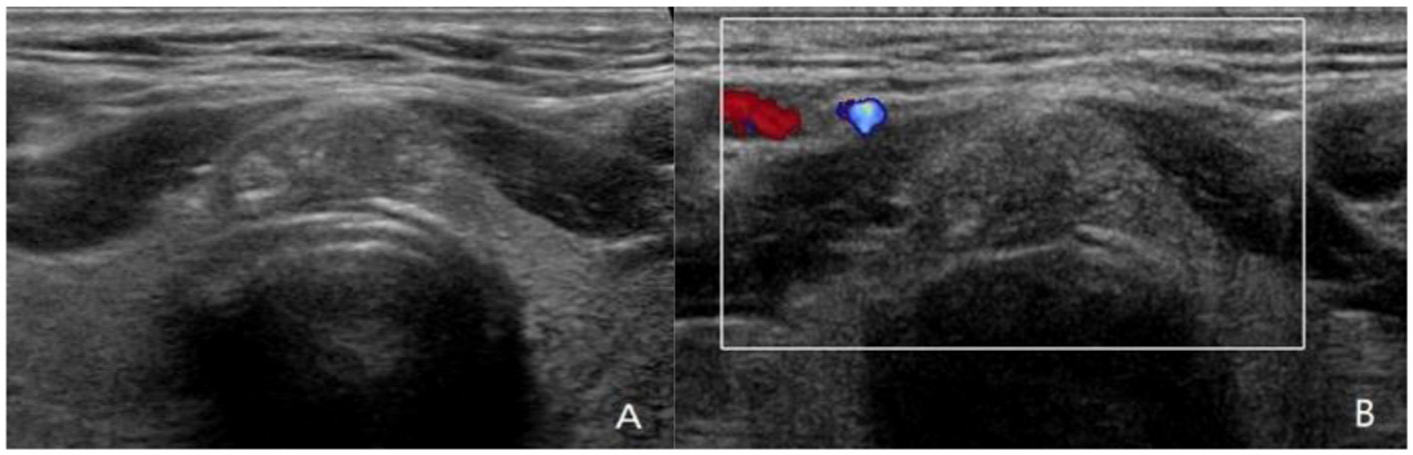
A 42-year-old man with papillary thyroid carcinoma (PTC) located in isthmus. (A,B) Ultrasound images show 1.3-cm solid nodule with hypoechoic, wider-than-tall, extrathyroidal extension (ETE), punctate echogenic foci, ACR TI-RADS 5. PTC was confirmed by fine-needle aspiration (FNA) cytologic examination before operation. The PTC in isthmus of thyroid was confirmed by postoperative pathology, with capsular invasion and bilateral paratracheal lymph node metastasis. Although ultrasound showed ETE, no abnormal lymph node was found.
